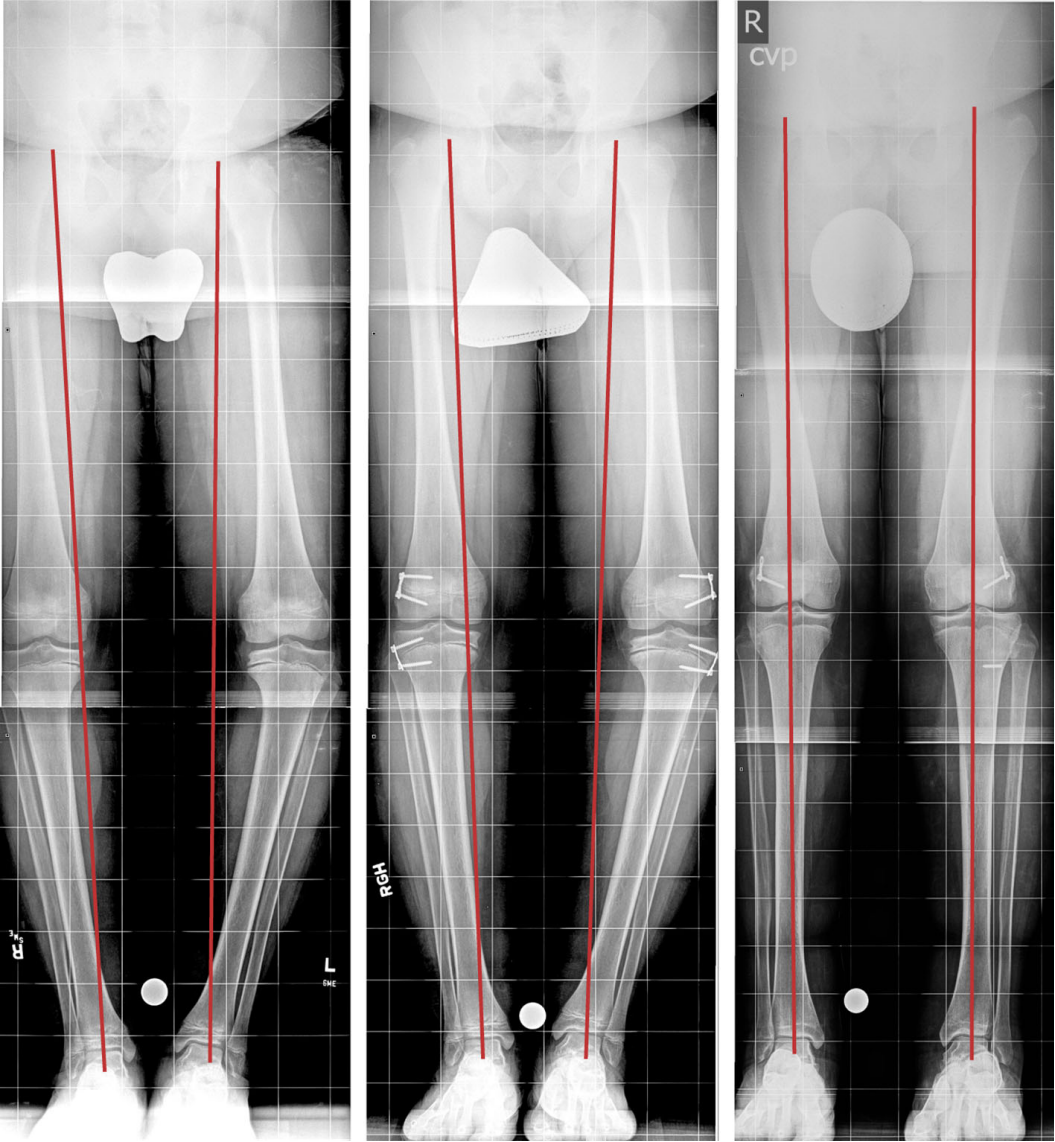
Twelve-year-old male with bilateral Blount disease, underwent bilateral distal femoral and proximal tibial lateral hemiepiphysiodsis with a TBP. a Long erect X-ray shows preoperative bilateral varus deformity with medial mechanical axis deviation, b 3 months after insertion of bilateral distal femur and proximal tibia lateral physeal TBP, c last follow-up with corrected deformity and central mechanical axis. The broken left tibial metaphyseal screw occurred 2 years earlier, when the first TBP was inserted for gradual varus correction. Copyright 2016, Rubin Institute for Advanced Orthopedics, Sinai Hospital of Baltimore
